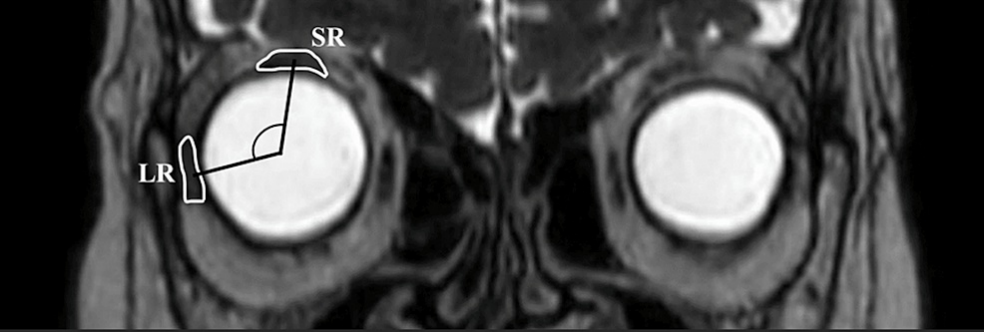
MRI image after the surgery After surgery, the dislocation angle improved to 104°.
